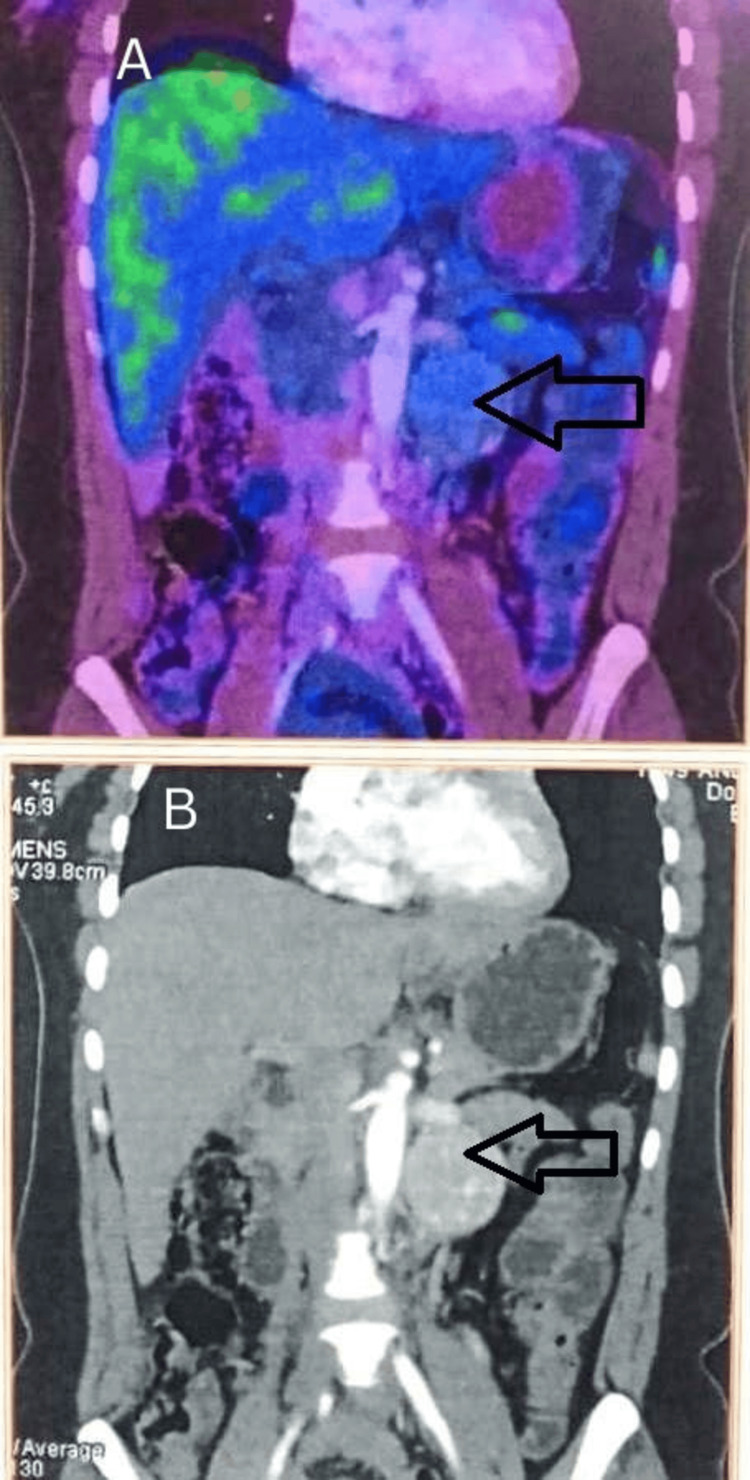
68Ga-DOTATATE PET/CT & contrast-enhanced CT of the abdomen. Panel A: Coronal section of 68Ga DOTATATE PET/CT shows very minimal uptake of the tracer by the lesion (black hollow arrow) in spite of very good contrast enhancement of the lesion located below the level of the left renal vein and lateral to the aorta in panel B (black hollow arrow).
